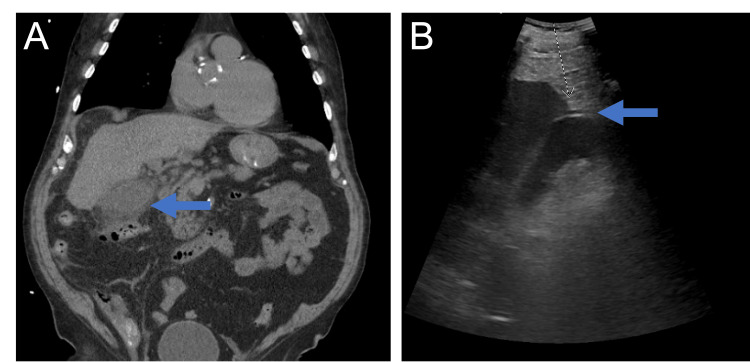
Computed Tomography (CT) of the Abdomen and Right Upper Quadrant (RUQ) Ultrasound Demonstrating Concern for Cholecystitis. Left: CT abdomen and pelvis showing distended gallbladder with wall thickening and pericholecystic inflammation. The arrow points to the inflamed gallbladder. Right: RUQ ultrasound showing evidence of cholelithiasis. The arrow points to a gallstone.
